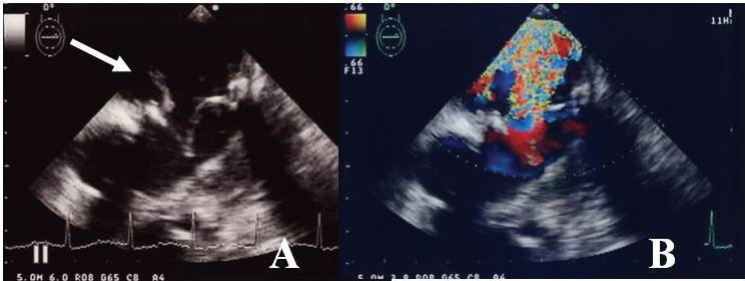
Echocardiography revealing prolapse of the Carpentier-Edwards Perimount bioprosthesis (white arrow) (A) and severe mitral regurgitation (B).
